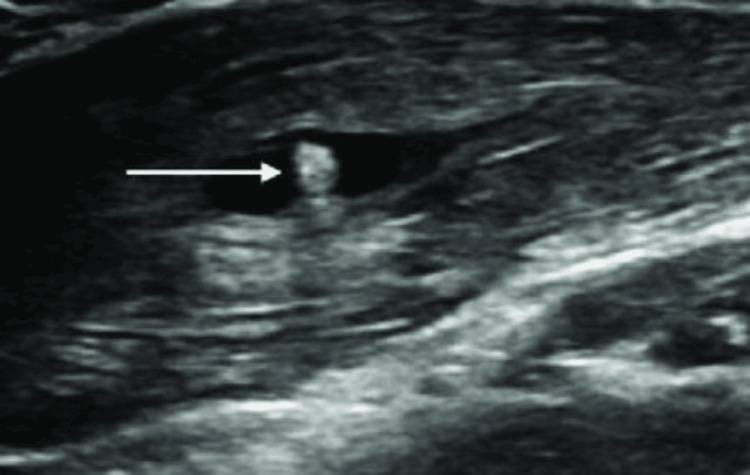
Ultrasonography image of the neck The white arrow shows scolex within the right sternocleidomastoid muscle
